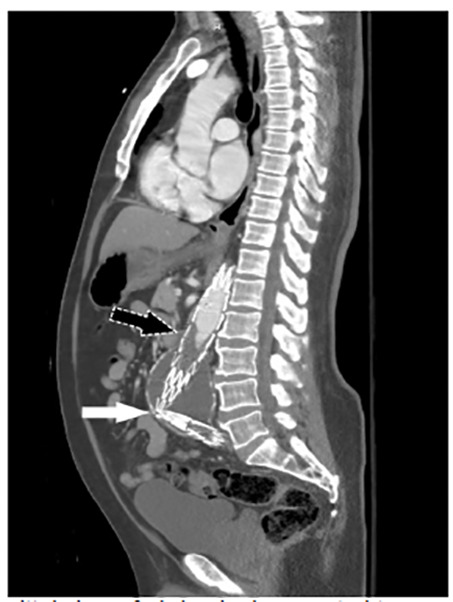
Sagittal view of abdominal computed tomography angiography demonstrating kinking of the aortic endograft (white arrow) and the presence of contrast having reached the location of the thrombus (black arrow) within the endograft.
